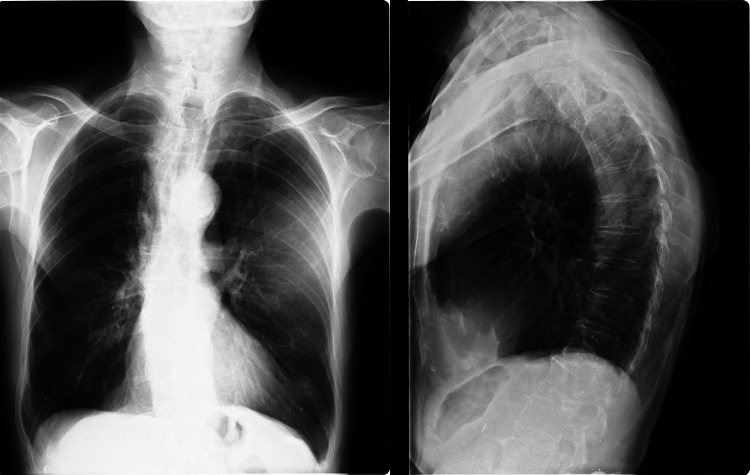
The space-occupying lesion on the posteroanterior and lateral chest radiograph.
